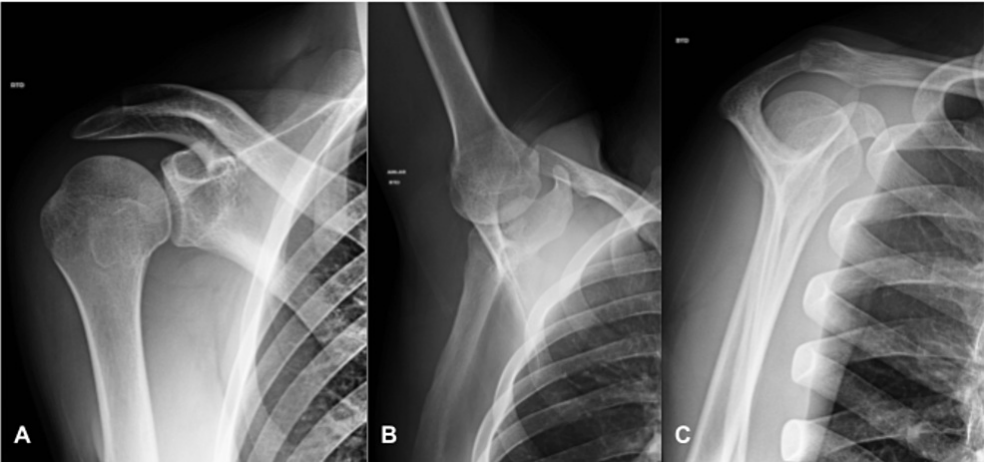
Post-reduction radiographic study of the right shoulder A - anteroposterior view; B - axillary view; C - Y view. All studies showed normal alignment of the bone structures, with no fractures or other bone lesions. No relevant soft tissue damage was apparent.
